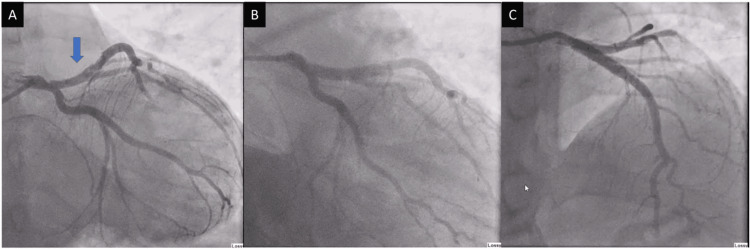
Emergent coronary angiogram. Emergent coronary angiogram demonstrated thrombotic plaque rupture at the ostium of the left anterior descending artery (LAD) with distal vessel occlusion (A) that was treated with IVUS-guided, deployed drug-eluting stent (DES) (B and C). IVUS: intravascular ultrasound
